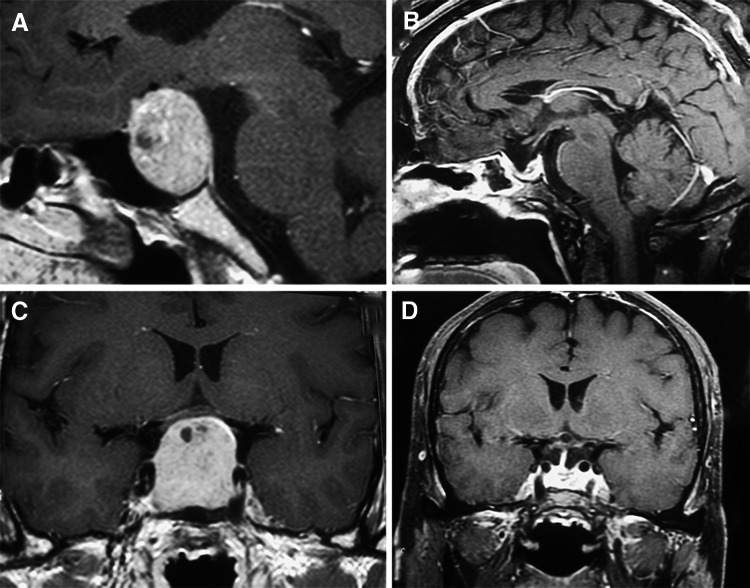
MRI images of one PRL adenoma. A, C Before surgery, B, D gross total resection after surgery
